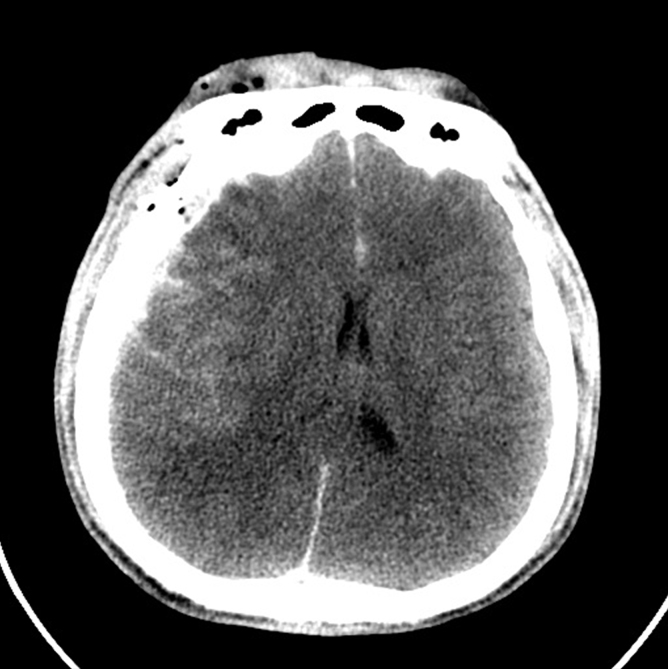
CT of the head before decompressive craniectomy shows right subdural hematoma and increased intracranial pressure with midline shift up to 8 mm. CT, computed tomography.
